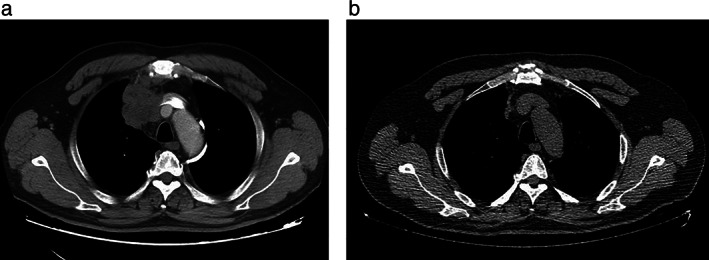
(a) Computed tomography (CT) from a male patient who presented with a primary mediastinal large B cell lymphoma. The long arrow shows the mediastinal mass. (b) CT scan from this patient after five cycles of the R‐CHOP regimen. The mediastinal mass decreased significantly
